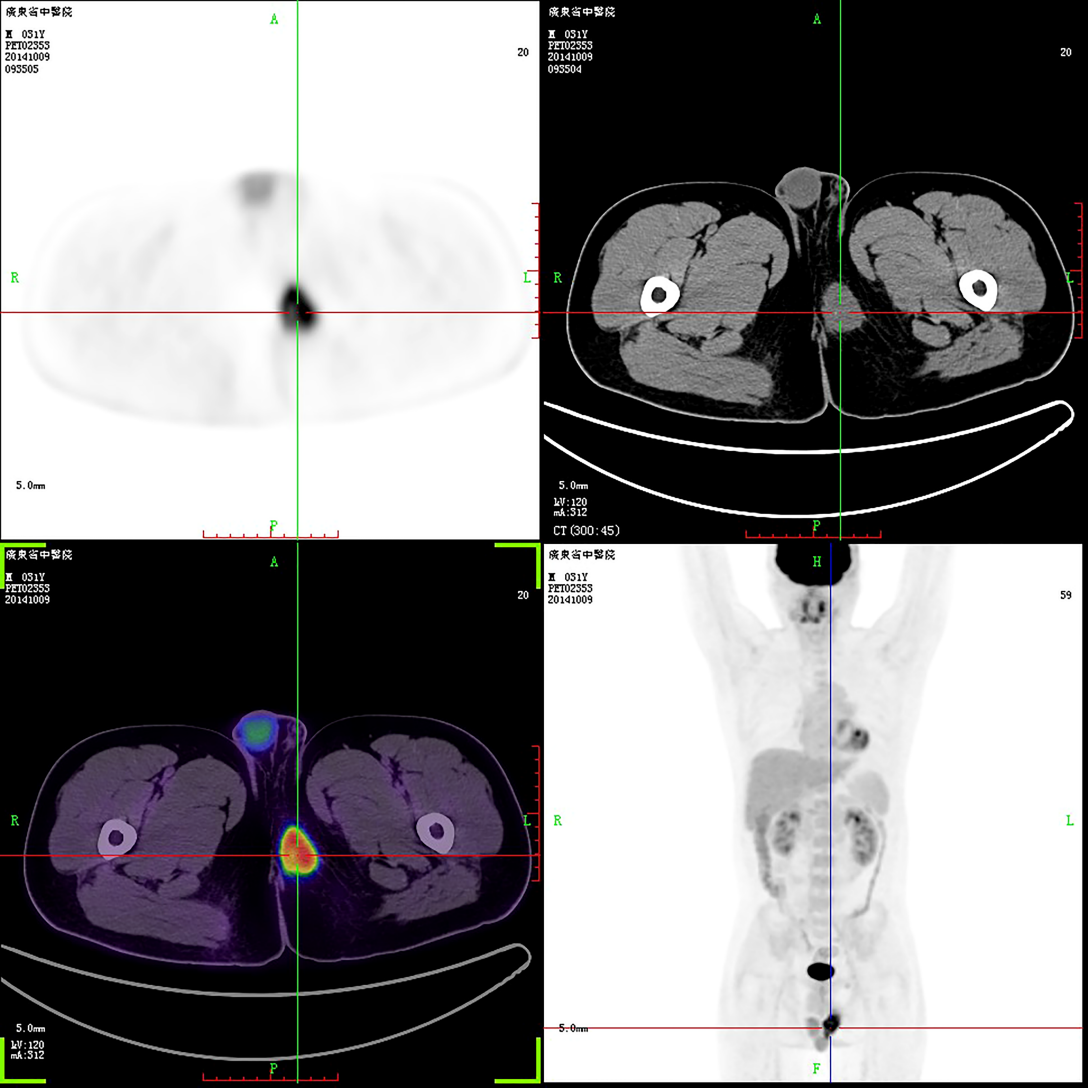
PET-CT revealed a perineal tumor. The purpose of uppercase letters is to mark the position.
